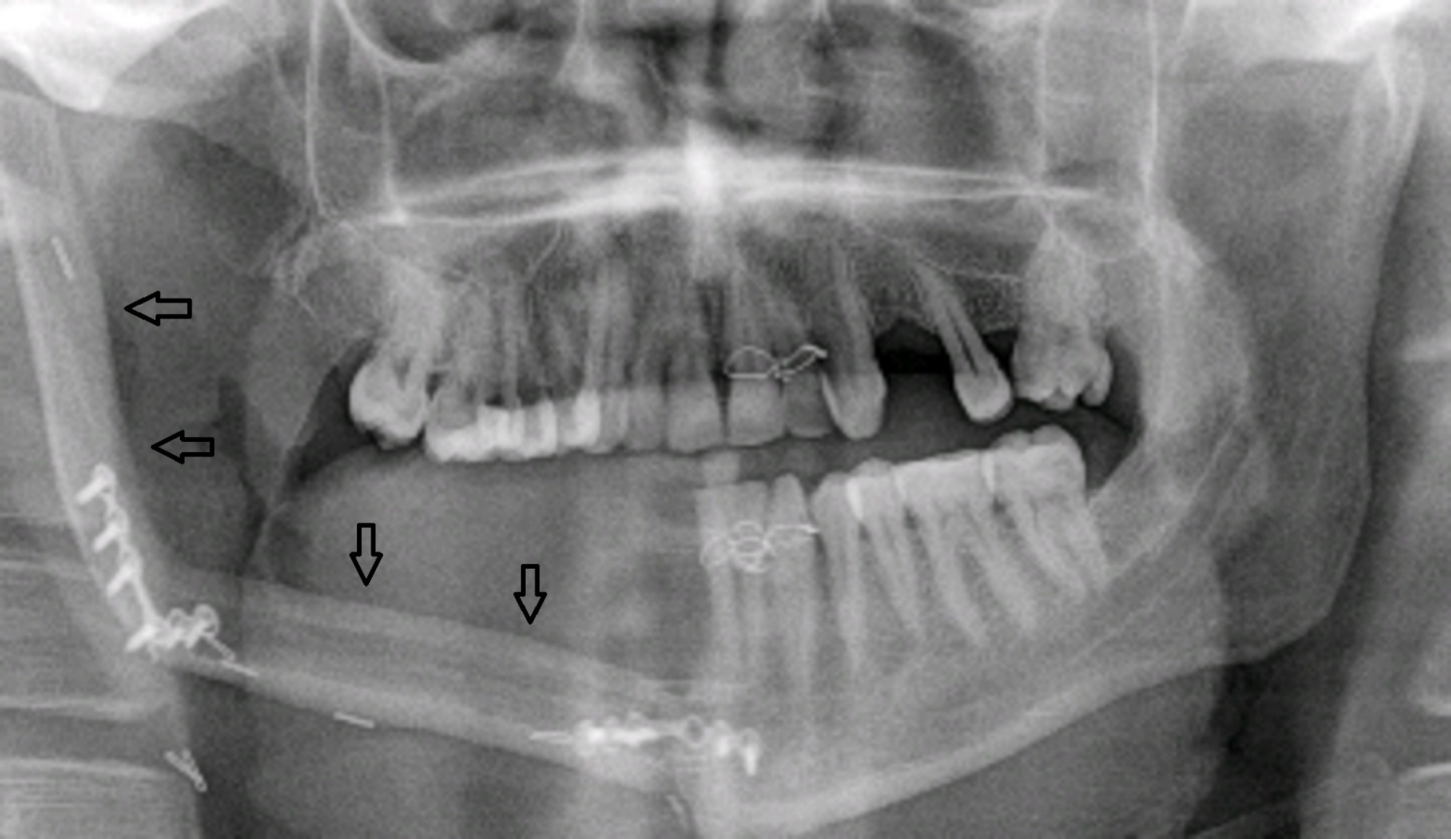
Patient 11. A 31-year-old male. Postoperative orthopantomogram shows position of free fibular flap (arrows).
